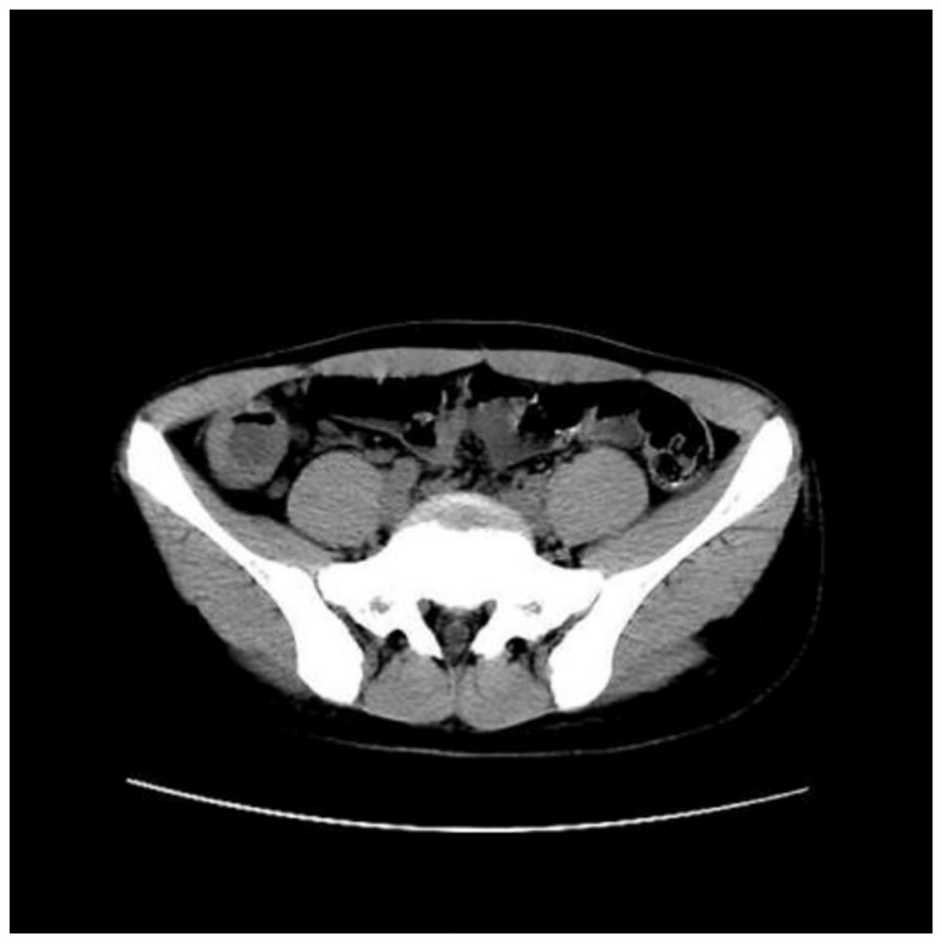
Abdominal CT scan showed obvious thickening of the ileocecal wall.
